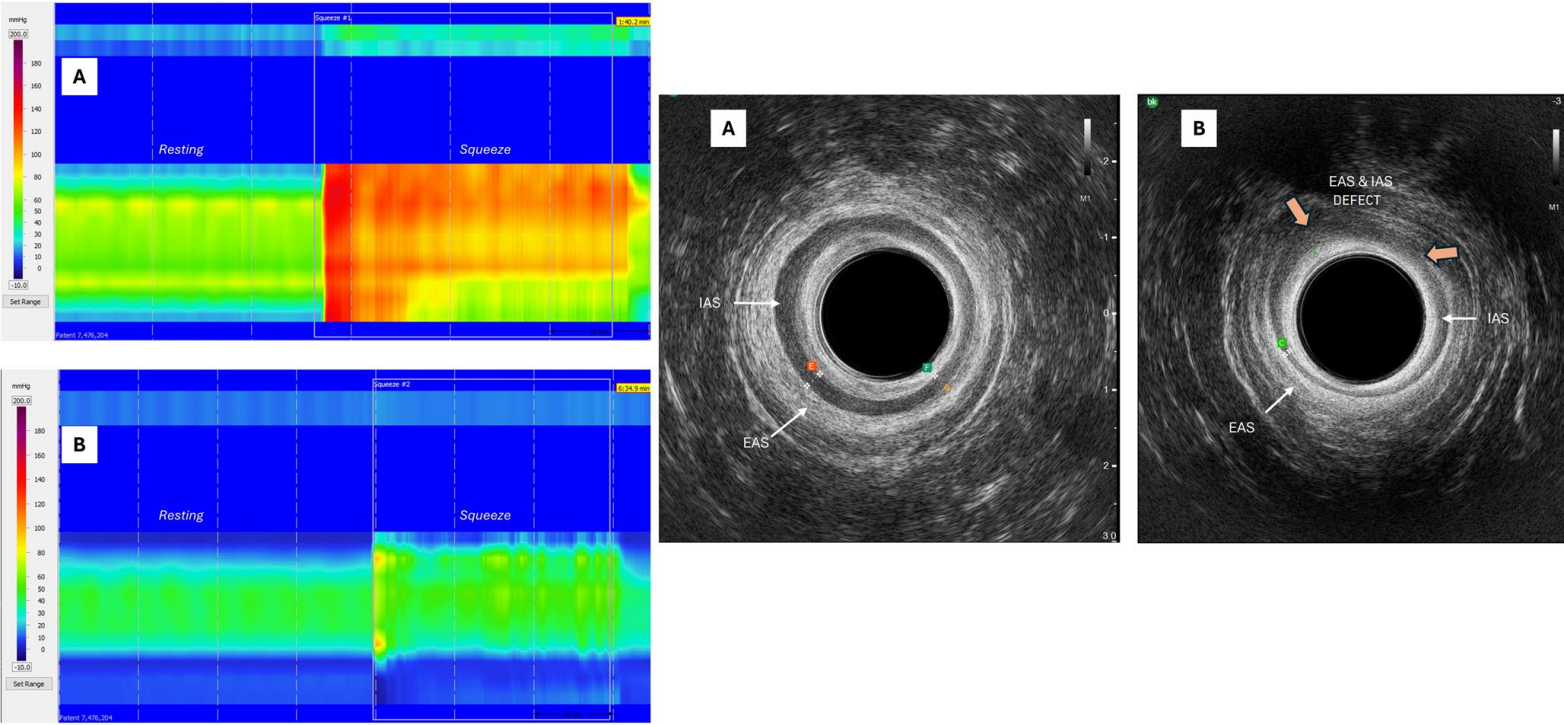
Left: High resolution anorectal manometry resting pressures and squeeze profile in a healthy patient (A) and a patient with fecal incontinence (B). Right: Anal ultrasound in a healthy patient (A) and patient with postpartum anal sphincter injury (B)
